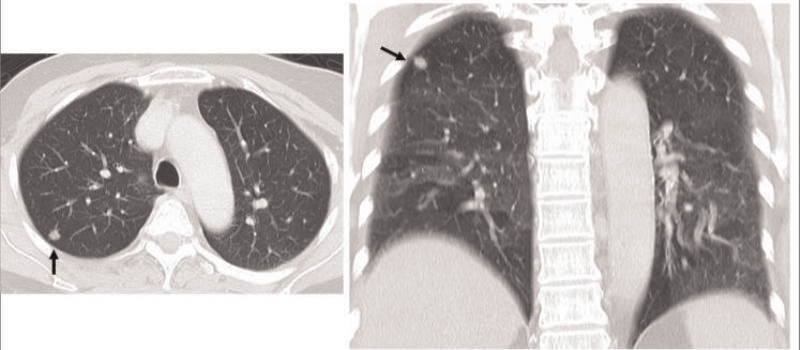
Chest X-ray and CT in Case 2 revealed a 0.8 cm nodular shadow over the B2 segment of the right upper pulmonary lobe.
